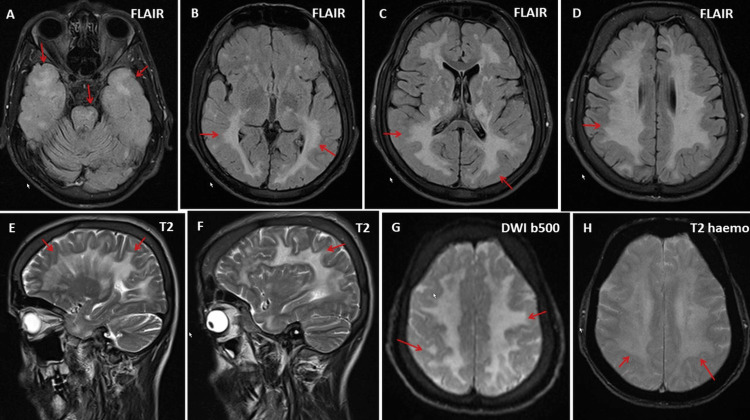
The MRI of the index patient shows extensive, supratentorial, patchy, confluent T2- and FLAIR-hyperintense areas (A-D). These are also hyperintense on DWI and T2 hemo-sequence (G-H). The basal ganglia are only minimally affected; the subcortical white matter is spared; a patchy pattern of involvement is also seen in the frontobasal and temporobasal regions. Additionally, small patchy, T2-hyperintense areas are found in the cerebellar peduncle and pons (E-F). The pons is atrophic, and there is evidence of incipient cerebellar atrophy MRI: magnetic resonance imaging; FLAIR: fluid-attenuated inversion recovery; DWI: diffusion-weighted imaging
